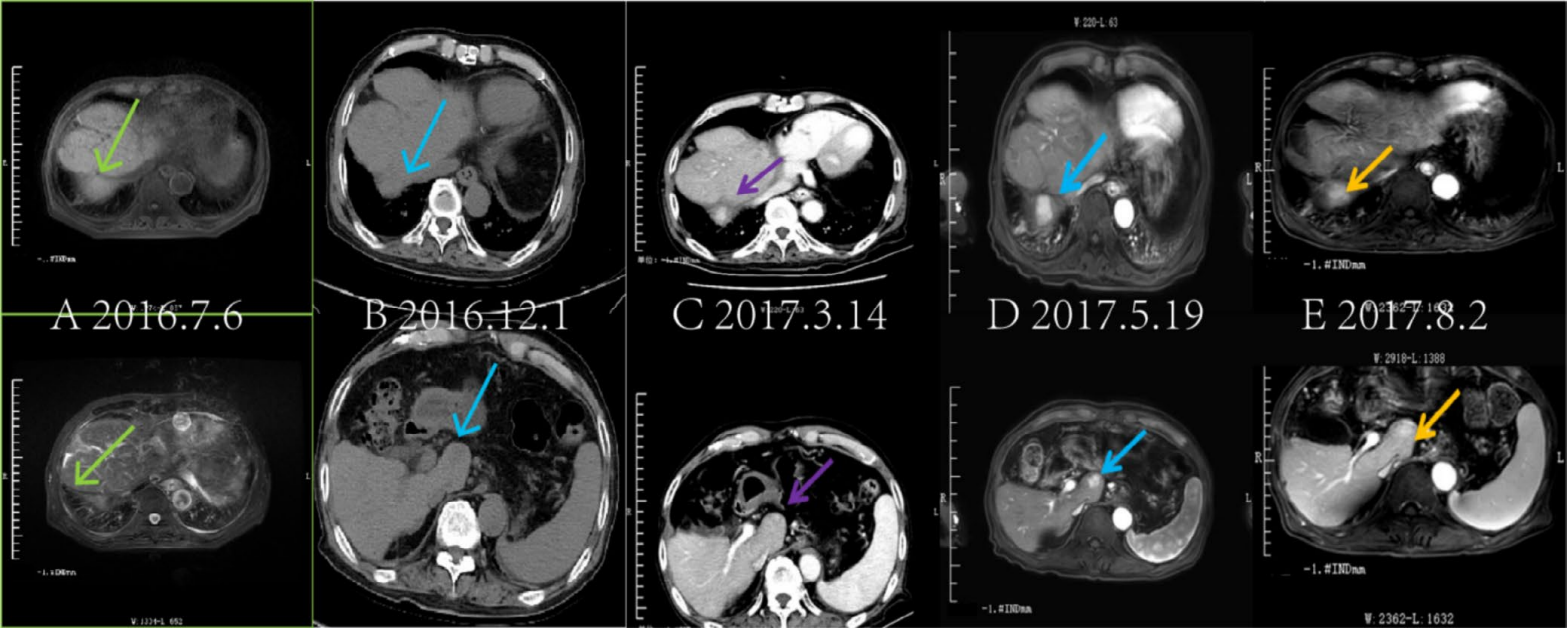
Changes in liver lesions between July 2016 and August 2017 revealed multiple abnormal enhancements in the superior posterior segment of the right and caudal lobes of the liver, which were greater than before. (A) The abdominal MRI shows the tumor‐occupying lesion in the right lobe of the liver. (B) The abdominal CT scan displays the tumor‐occupying lesion in the caudal lobes of the liver. (C) The abdominal enhanced CT scan demonstrates the liver lesions more clearly. (D, E) The abdominal MRI reveals an increase in liver mass compared to before (arrows).
